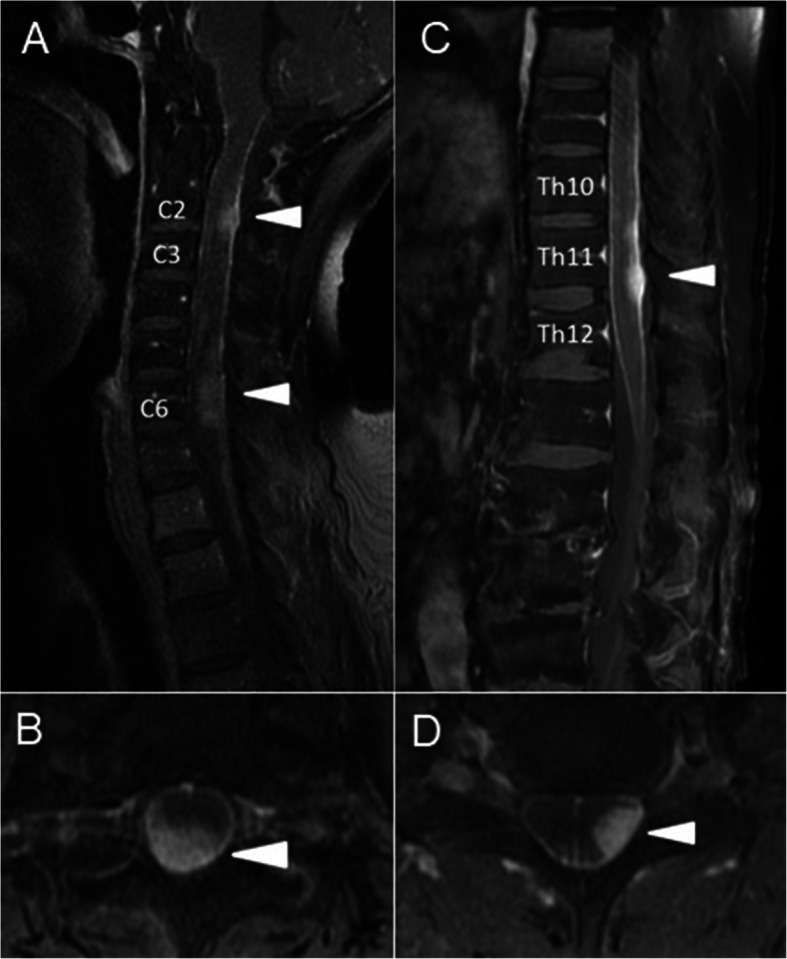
T1-weighted magnetic resonance image with gadolinium on admission showing multiple contrast-enhanced lesions in the posterior intramedullary spinal cord at the C2 and C6 levels and on the spinal cord surface at the C7 and C8 levels (A, B), and in the left posterior intramedullary spinal cord at the Th12 level and on the surface of the spinal cone (C, D)
